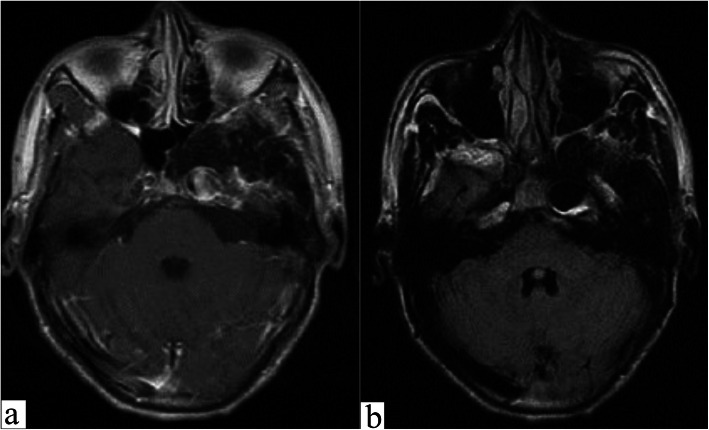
Magnetic resonance imaging shows an aneurysm of the left internal carotid artery. a Enhanced scanning shows mild uneven enhancement and heterogeneous signal intensities representing variable stages of thrombosis. b T2 magnetic resonance imaging shows aneurysm is low signal and blood vessel wall calcification
